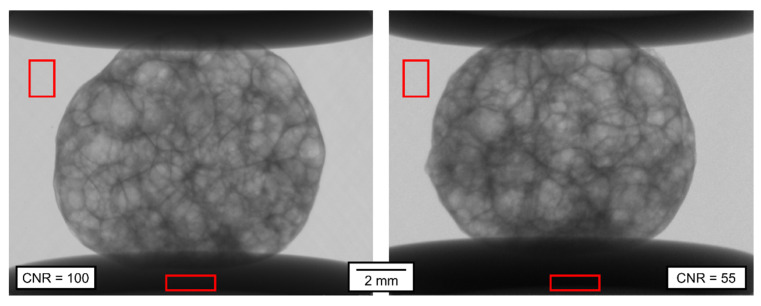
Comparison of the radiographic projections of the APM foam elements. Left: Mode B; Right: Mode D. ROIs for CNR evaluation indicated in red.
